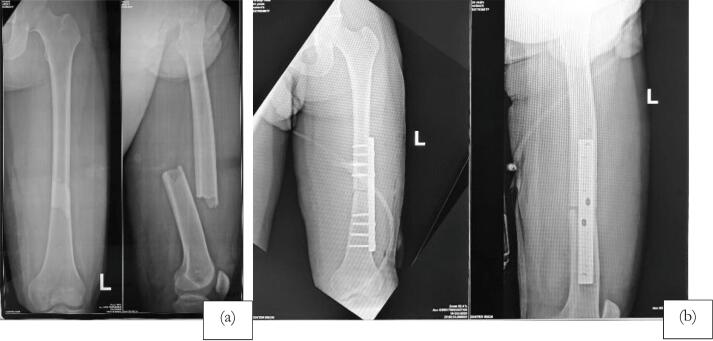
(a) Preoperative X-ray demonstrated fracture of the left femoral shaft. (b) Initial radiological presentation and post-operative x-ray with plate fixation.
